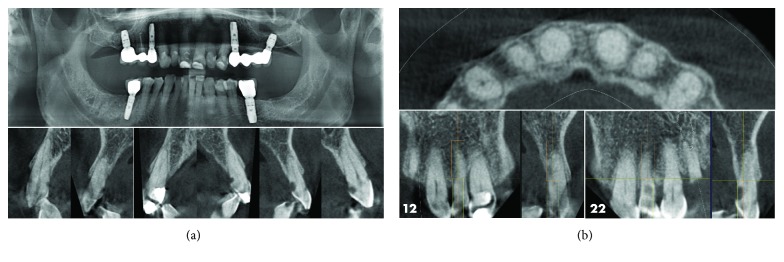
(a) Panoramic X-ray. (b) 3D slices showing the implant planning. The root caries can be seen.
